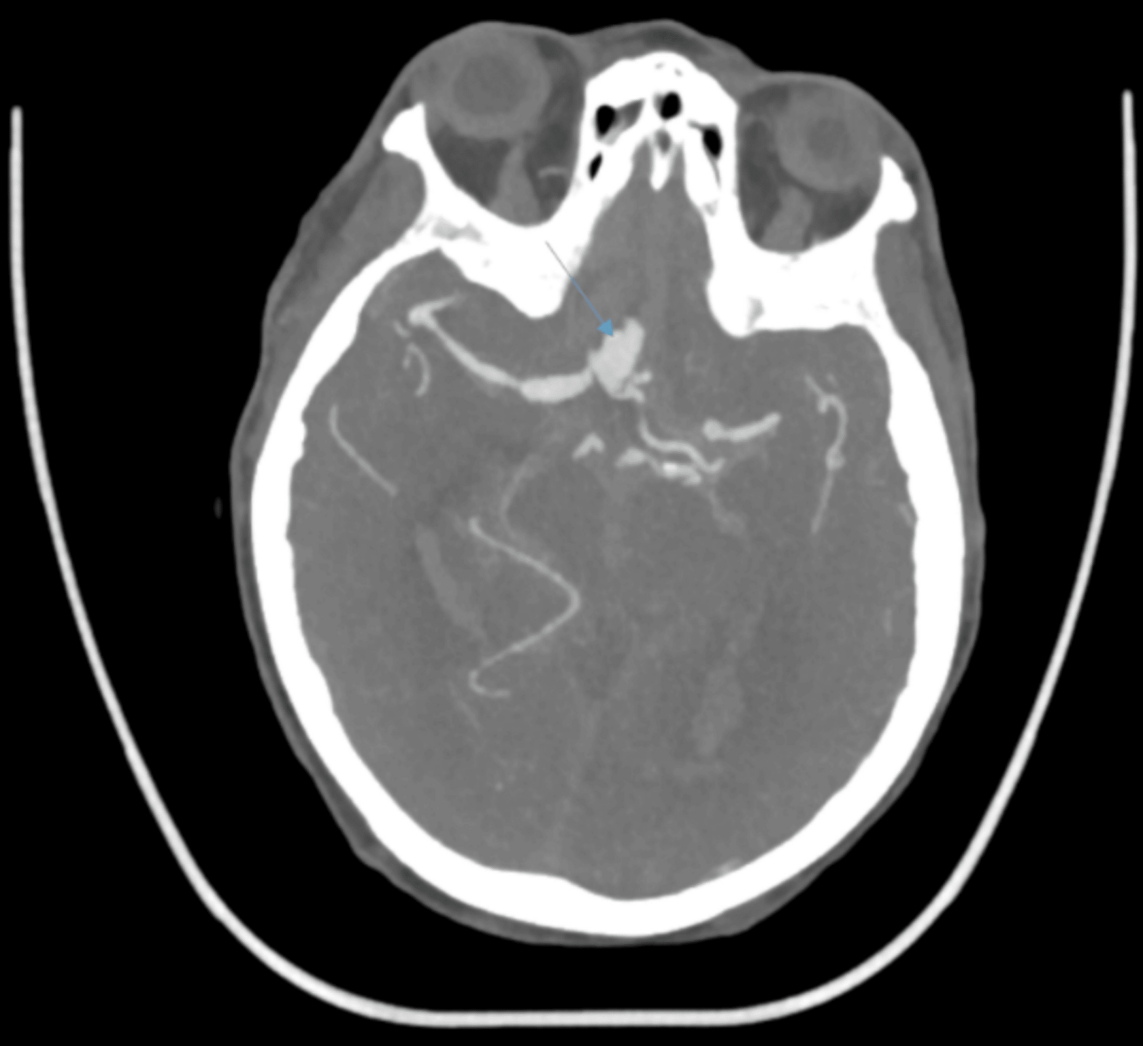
Axial section CTA showing an irregular outline of the anterior communicating artery aneurysm.
